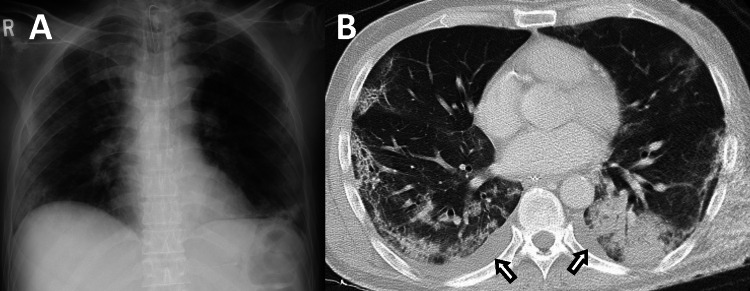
Chest X-ray and computed tomography on day 4 (A) Chest X-ray on day 4 showed bilateral peripheral consolidation. (B) Chest computed tomography on the same day also showed peripheral lung consolidation predominant on dorsal distribution. Pleural effusions were also seen (arrow).
